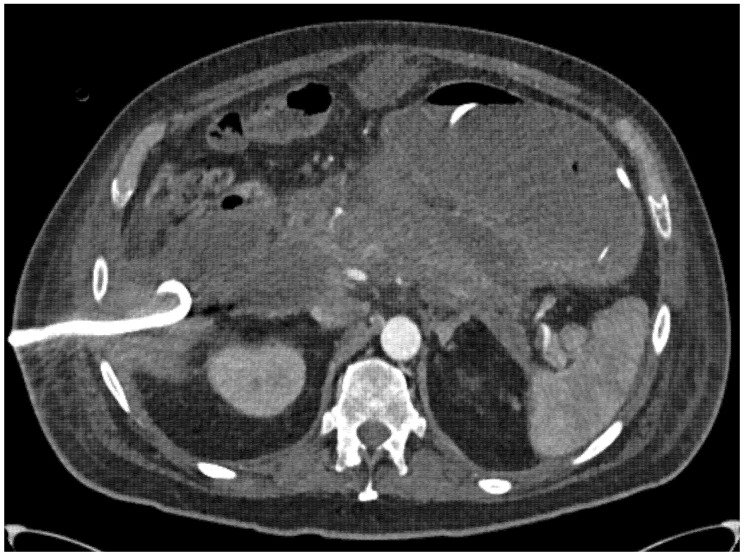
CT-guided drainage of the necrotic cavity.
